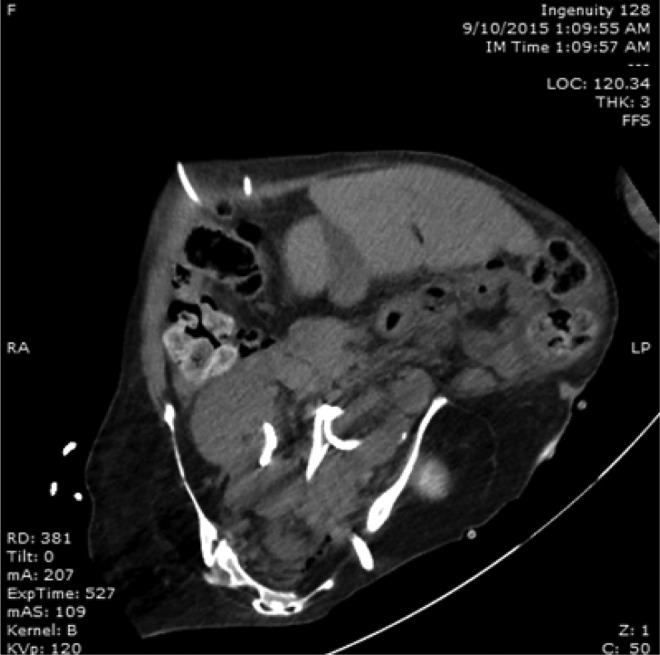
Postoperative CT imaging following primary PCNL. Left nephrostomy tube can be seen entering through the sciatic foramen. A portion of right nephrostomy tube visible in the right renal pelvis.
